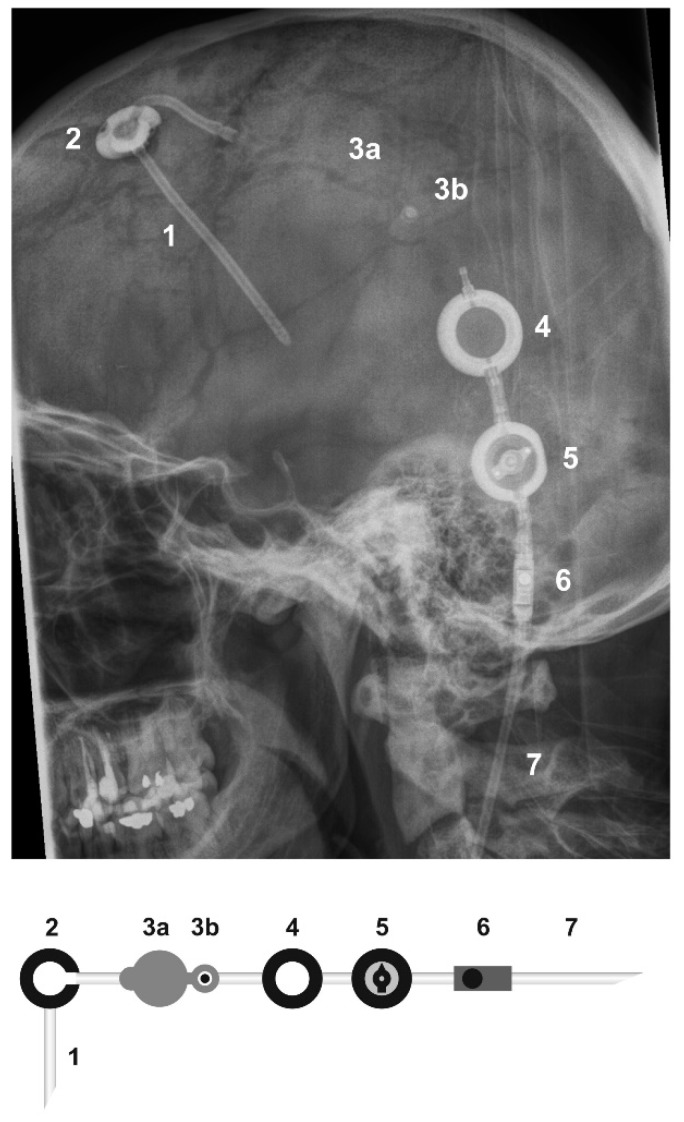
Radiography immediately after shunt implantation in patient 1 and scheme depicting the arrangement of the shunt components. 1 = proximal ventricular catheter; 2 = Rickham reservoir; 3a = on-off valve; 3b = button of the on-off valve; 4 = control reservoir; 5 = programmable differential pressure unit; 6 = gravitational unit; 7 = distal peritoneal catheter.
